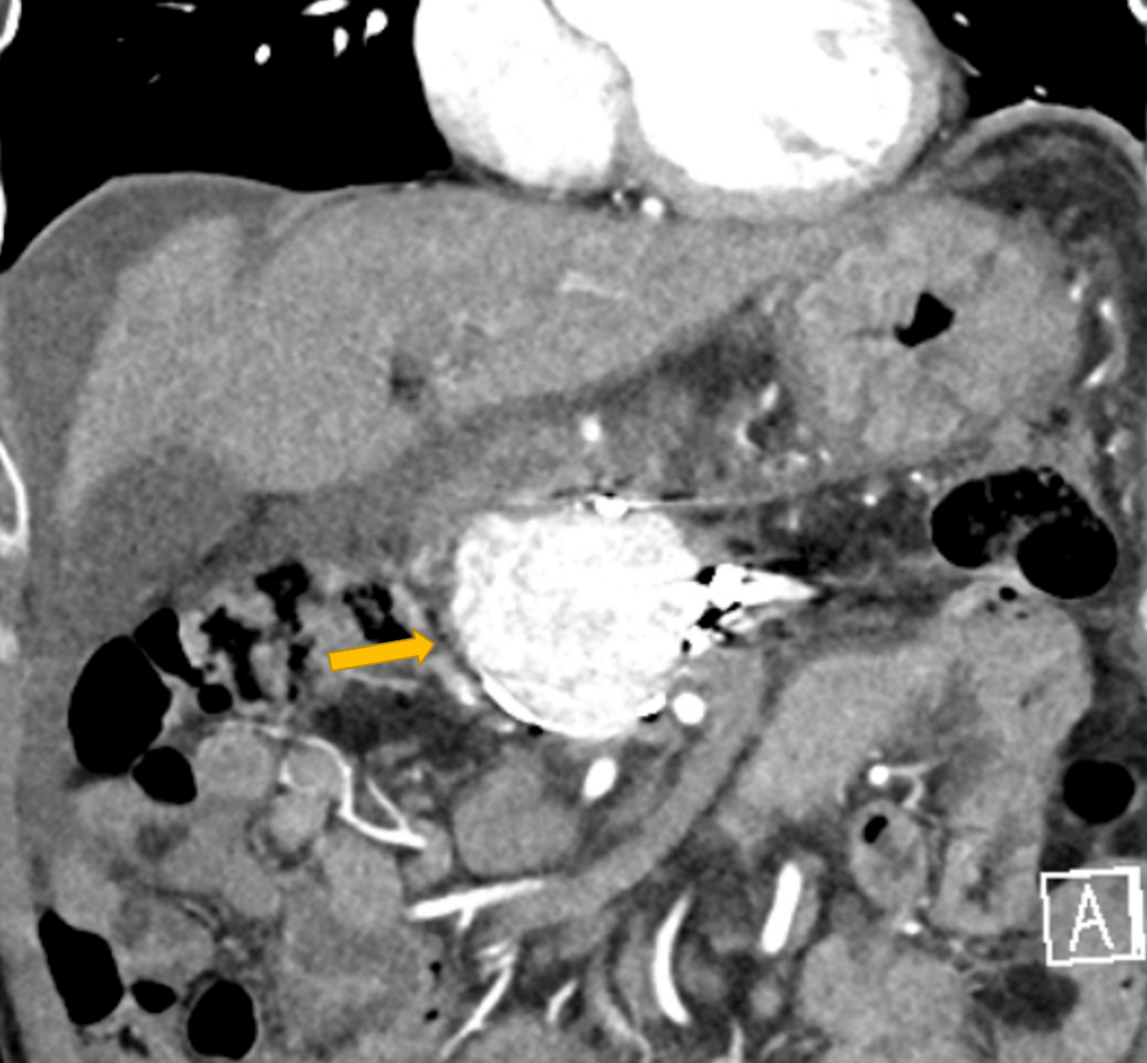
Haemorrhagic PP managed with multi-session angiographic embolization to achieve haemostasis. The PP (yellow arrow) is partially embolised but still filling up with contrast on CT. PP: Pancreatic Pseudocyst; CT: computed tomography
